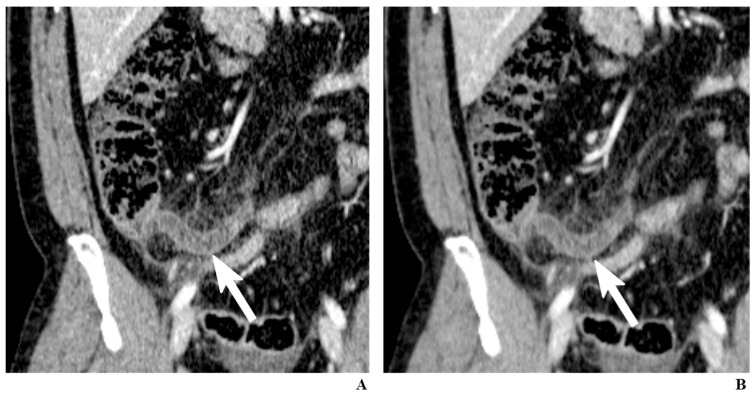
A 34-year-old man with appendicitis. Contrast-enhanced coronal CT images reconstructed by filtered back-projection (FBP) (A) and iterative reconstruction (IR) (B). While both images clearly depict an inflamed appendix (arrows), the image reconstructed using IR generates less noise, which can alleviate the practitioners’ reluctance to low-dose CT.
